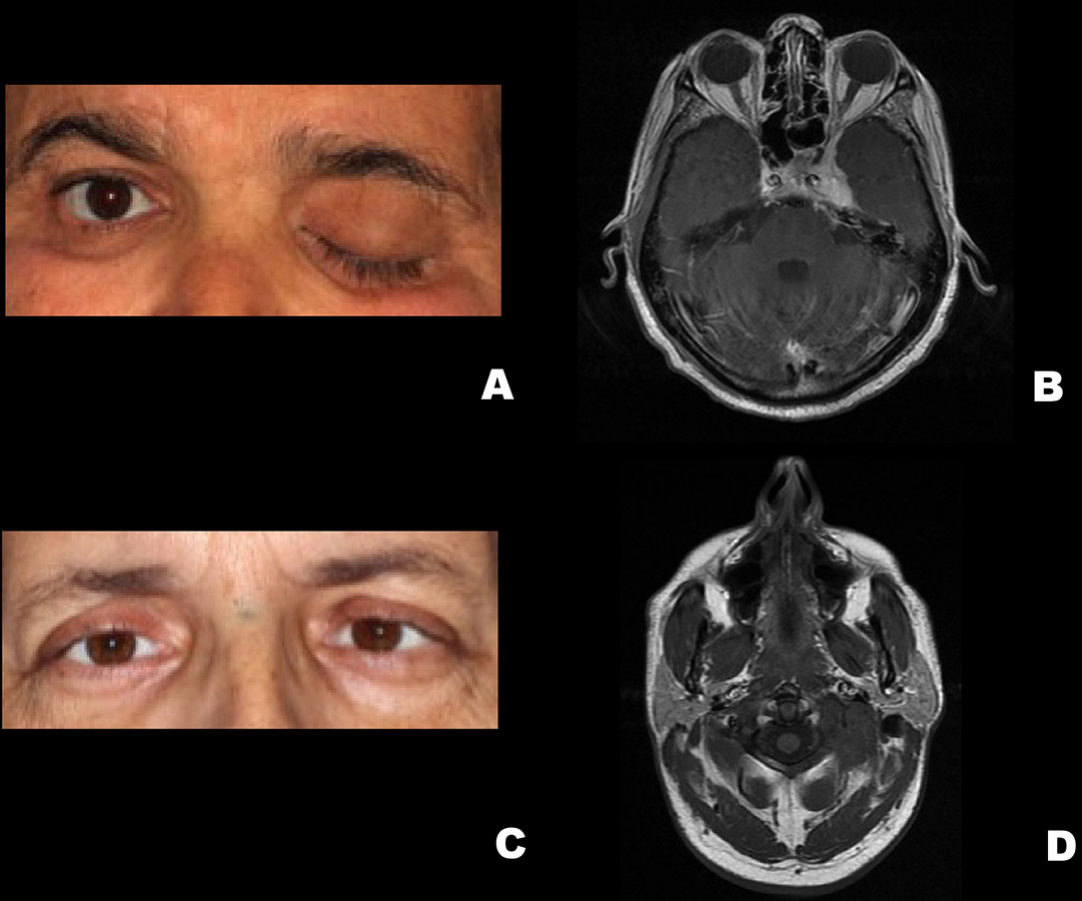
Patient 3. A. Extraoral clinical aspect showing left eyelid ptosis. B. Axial IMR (T2-weighted) demonstrating hyperintense image at the skull base causing enlargement of the left optical canal. Patient 4. C. Extraoral clinical aspect revealing fixed globe in the left eye. D. Axial IMR (T2-weighted) demonstrating a mass involving the left sphenoid bone and causing hypoglossal canal obliteration.
